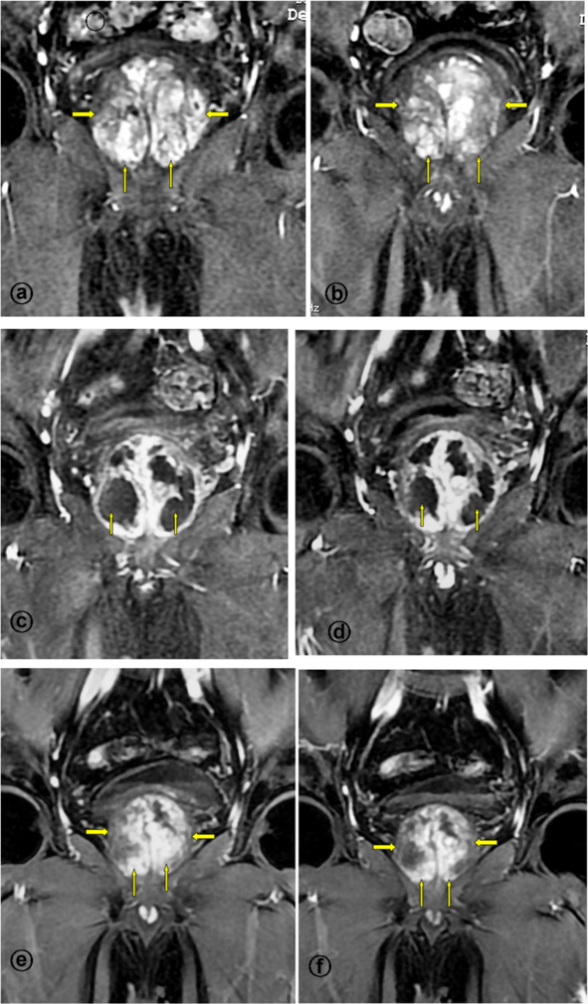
MR Images from a patient with lower urinary tract symptoms due to large benign prostatic hyperplasia underwent bilateral PAE, the same case as the Figure 4. a-b. Enhanced T1-weighted coronal MR images obtained before PAE shows a large benign prostatic hyperplasia (straight arrows). c-d. Enhanced T1-weighted coronal MR images obtained at 1-month after PAE shows significantly infarct areas on the both side of the prostate (straight arrows), with the volume reduction of 12%. e-f. Enhanced T1-weighted coronal MR images obtained at 12-month after PAE shows the prostate volume reduction of 62%; this patient experienced marked clinical improvement during 32 months follow-up, with IPSS improvement of 85%.
